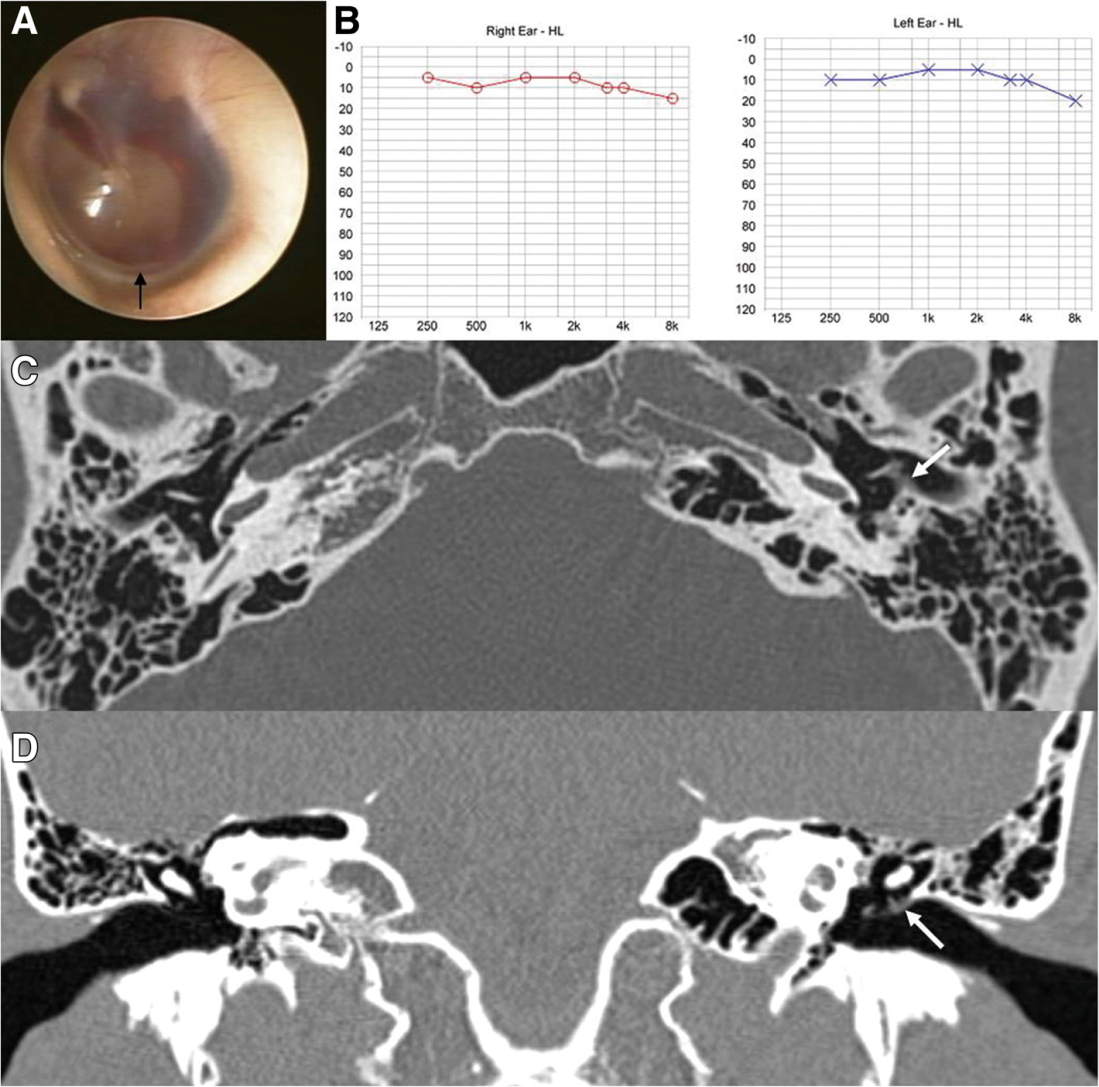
Otoendoscopic examination (a) of patient 2 revealed a small hemorrhage behind the intact left tympanic membrane (TM) and along the line between the tympanic annulus and epidermal layer of the TM (black arrow). Initial pure tone audiometry showed normal hearing on the left side (b), and follow-up pure tone audiometry after 1 week revealed no change (c). Temporal bone computed tomography demonstrated mild thickening of the left TM (white arrows) on axial (d) and coronal views (e), suggesting iTM hemorrhage
